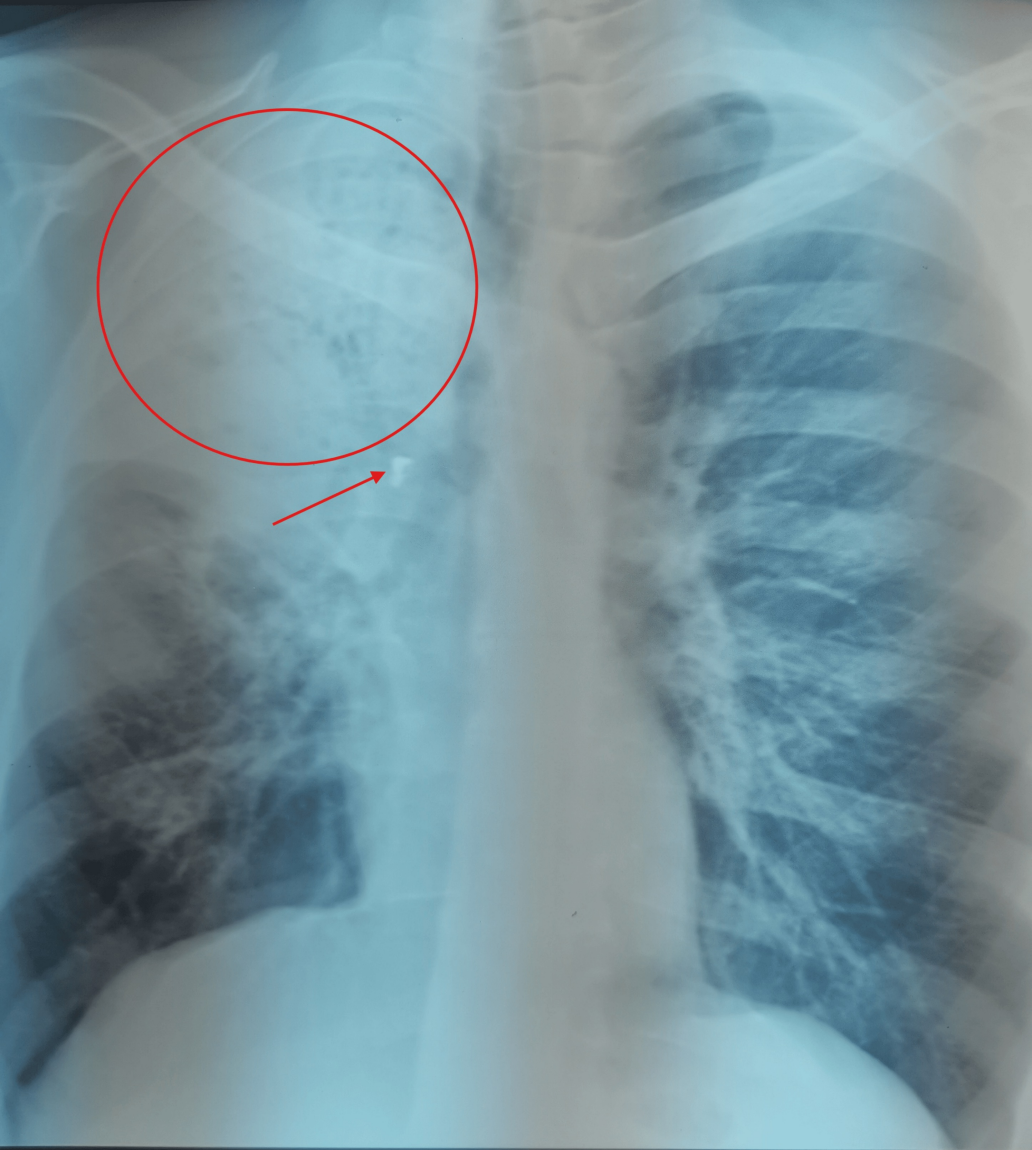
Frontal chest X-ray showing acute lobar pneumonia (red circle) with a calcified opacity projected over the right upper lobe bronchial orifice (red arrow).
